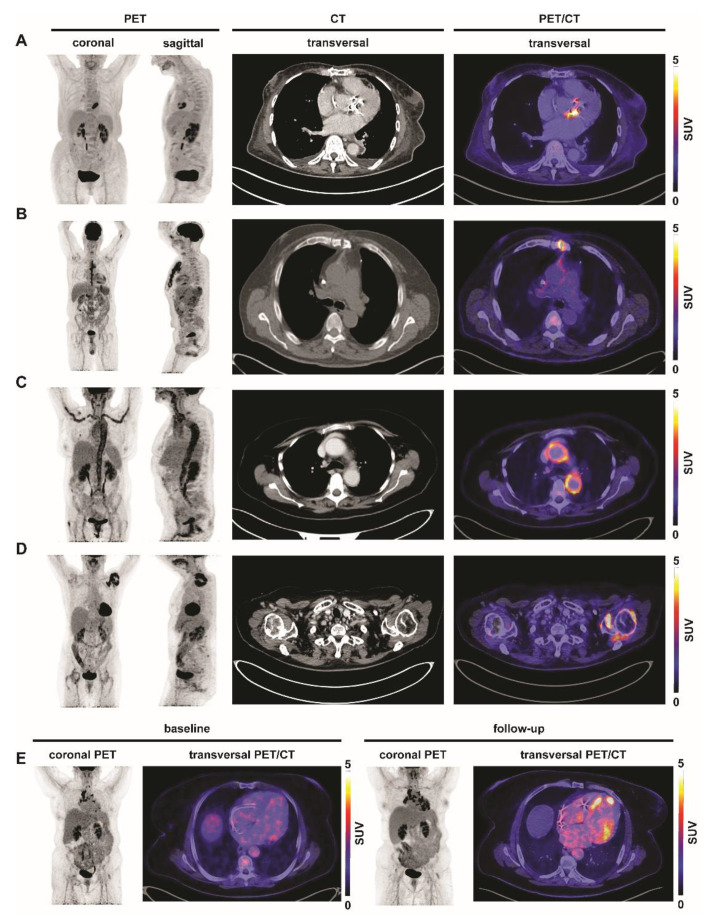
Inflammation imaging with ^18^F-FDG in various inflammatory conditions. Representative coronal, sagittal and transversal ^18^F-FDG PET/CT images of patients with different inflammatory conditions. (A) Aortic valve abscess. Patient received aortic valve replacement. Echocardiographic suspicion for infected aortic valve was confirmed by ^18^F-FDG-PET after dietary preparation which revealed circular tracer uptake around the aortic valve reported as aortic valve abscess. (B) Fever of unknown origin – osteomyelitis. Patient with fever of unknown origin who underwent sternotomy three months prior imaging. ^18^F-FDG uptake can be seen along the sternum supporting clinical suspicion of osteomyelitis. (C) Fever of unknown origin—arteritis. Patient with recurrent fever of unknown origin for three months and increasing c-reactive protein levels. ^18^F-FDG-PET shows inflammation of the large vessels consistent with a Takayasu’s arteritis. (D) Polyarthritis. Follow-up ^18^F-FDG-PET of a patient with malignant melanoma and polyarthritis with progressive pain. PET shows active arthritis in the left shoulder. (E) Sarcoidosis. Patient with suspicion of progressive sarcoidosis and newly diagnosed cardiac arrhythmia. ^18^F-FDG-PET after dietary preparation show increased uptake in bihilary lymph nodes at baseline. Follow-up ^18^F-FDG PET/CT shows increased inflammatory activity in bihilary lymph nodes and further myocardial involvement. SUV, standardized uptake value.
